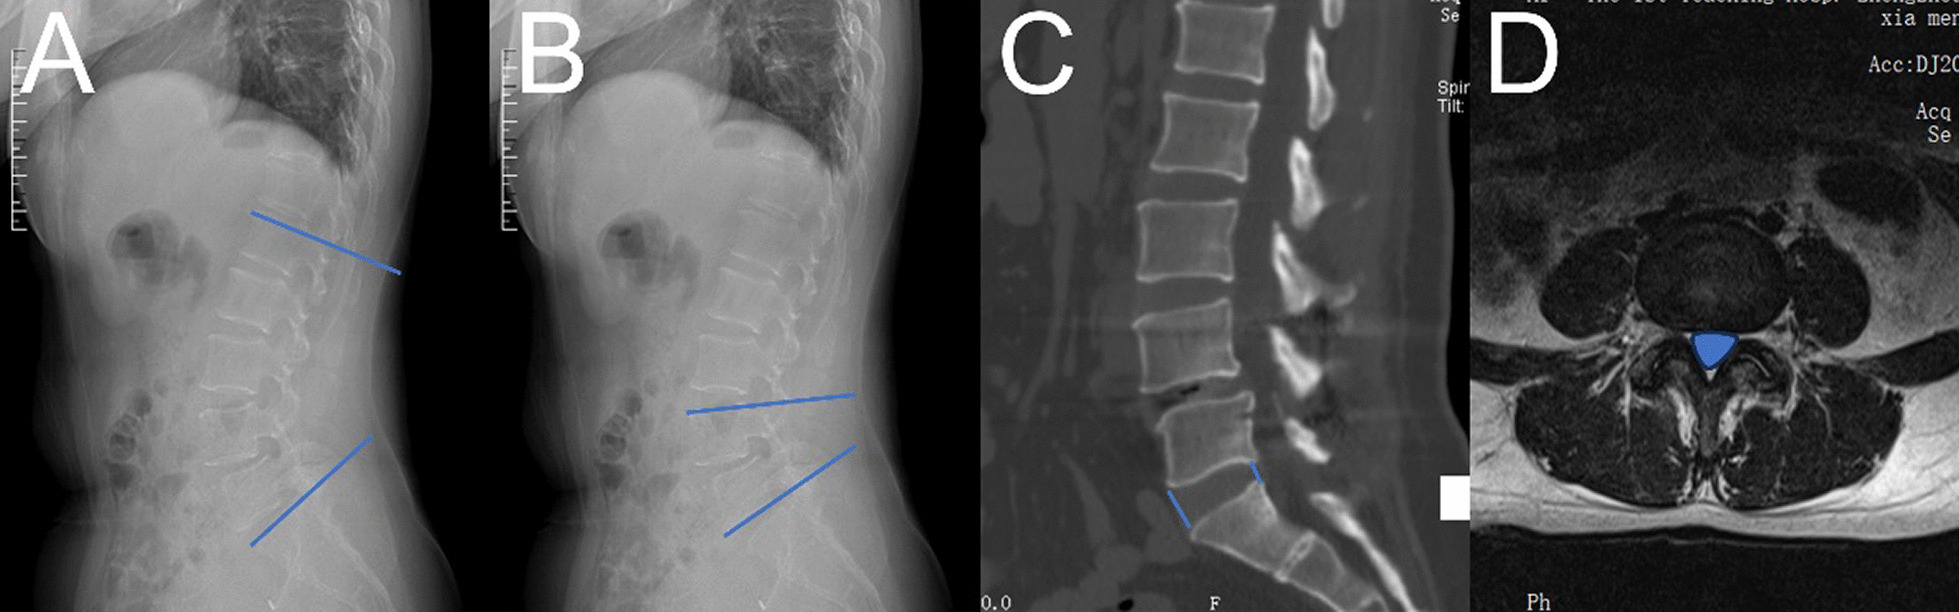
The measurement technique for each Radiological indicators. A Measurement of LL on lateral roentgenograph; B Measurement of Segmental angle on lateral roentgenograph; C Measurement of DH on sagittal CT; D Measurement of CSA of spinal canal on axial MRI
